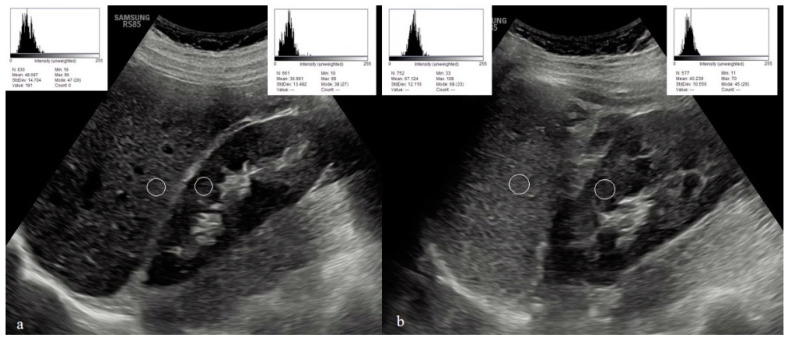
Hepatic/renal (a), and splenic/renal (b) echointensity measurements. The white circles correspond to the regions of interest used in the ultrasound analyses.
